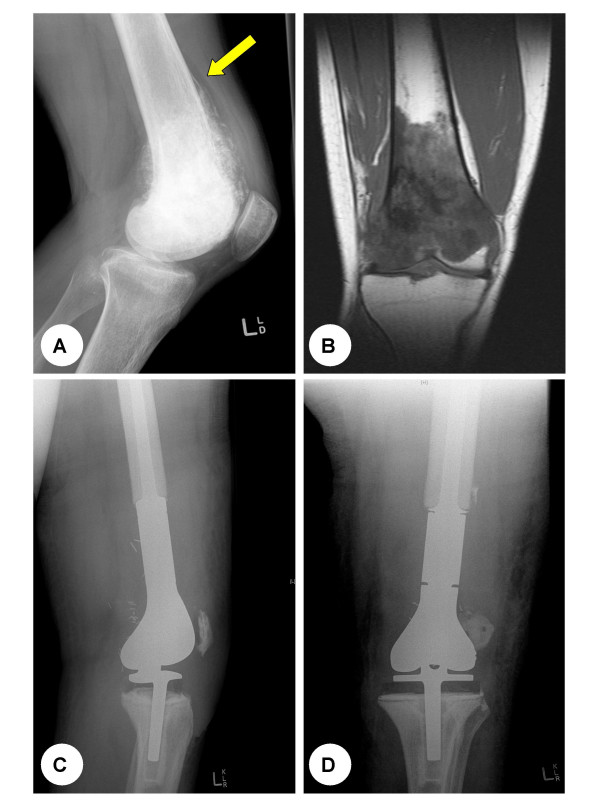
(A) Plain lateral radiograph showing the characteristic ill-defined lesion with both lytic and osteoblastic features and evidence of periosteal elevation (Codman's triangle) (arrow). (B) T1-weighted magnetic resonance image showing the intramedullary extension of the tumour, with cortical destruction and extension into the soft tissue. (C) Lateral and (D) anteroposterior plain radiographs post distal femoral resection and reconstruction of the knee joint with a GMRS (Global modular replacement system, Stryker Howedica) megaprosthesis.
